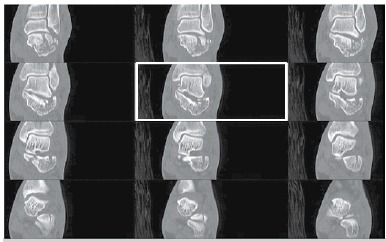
Example of assembly with 12 sequential coronal CT slices of patients with calcaneal fractures present in Power Point files used for classification by observers.
